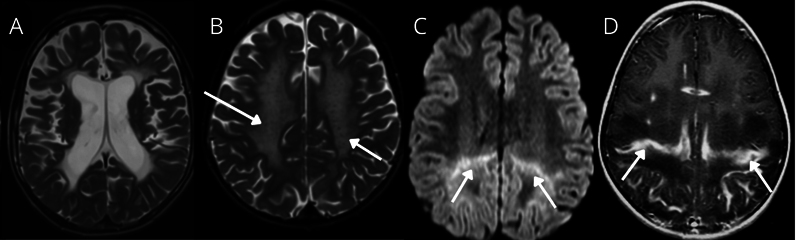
Abbreviations: DWI, diffusion-weighted imaging; MLD, metachromatic leukodystrophy; X-ALD, X-linked adrenoleukodystrophy.
